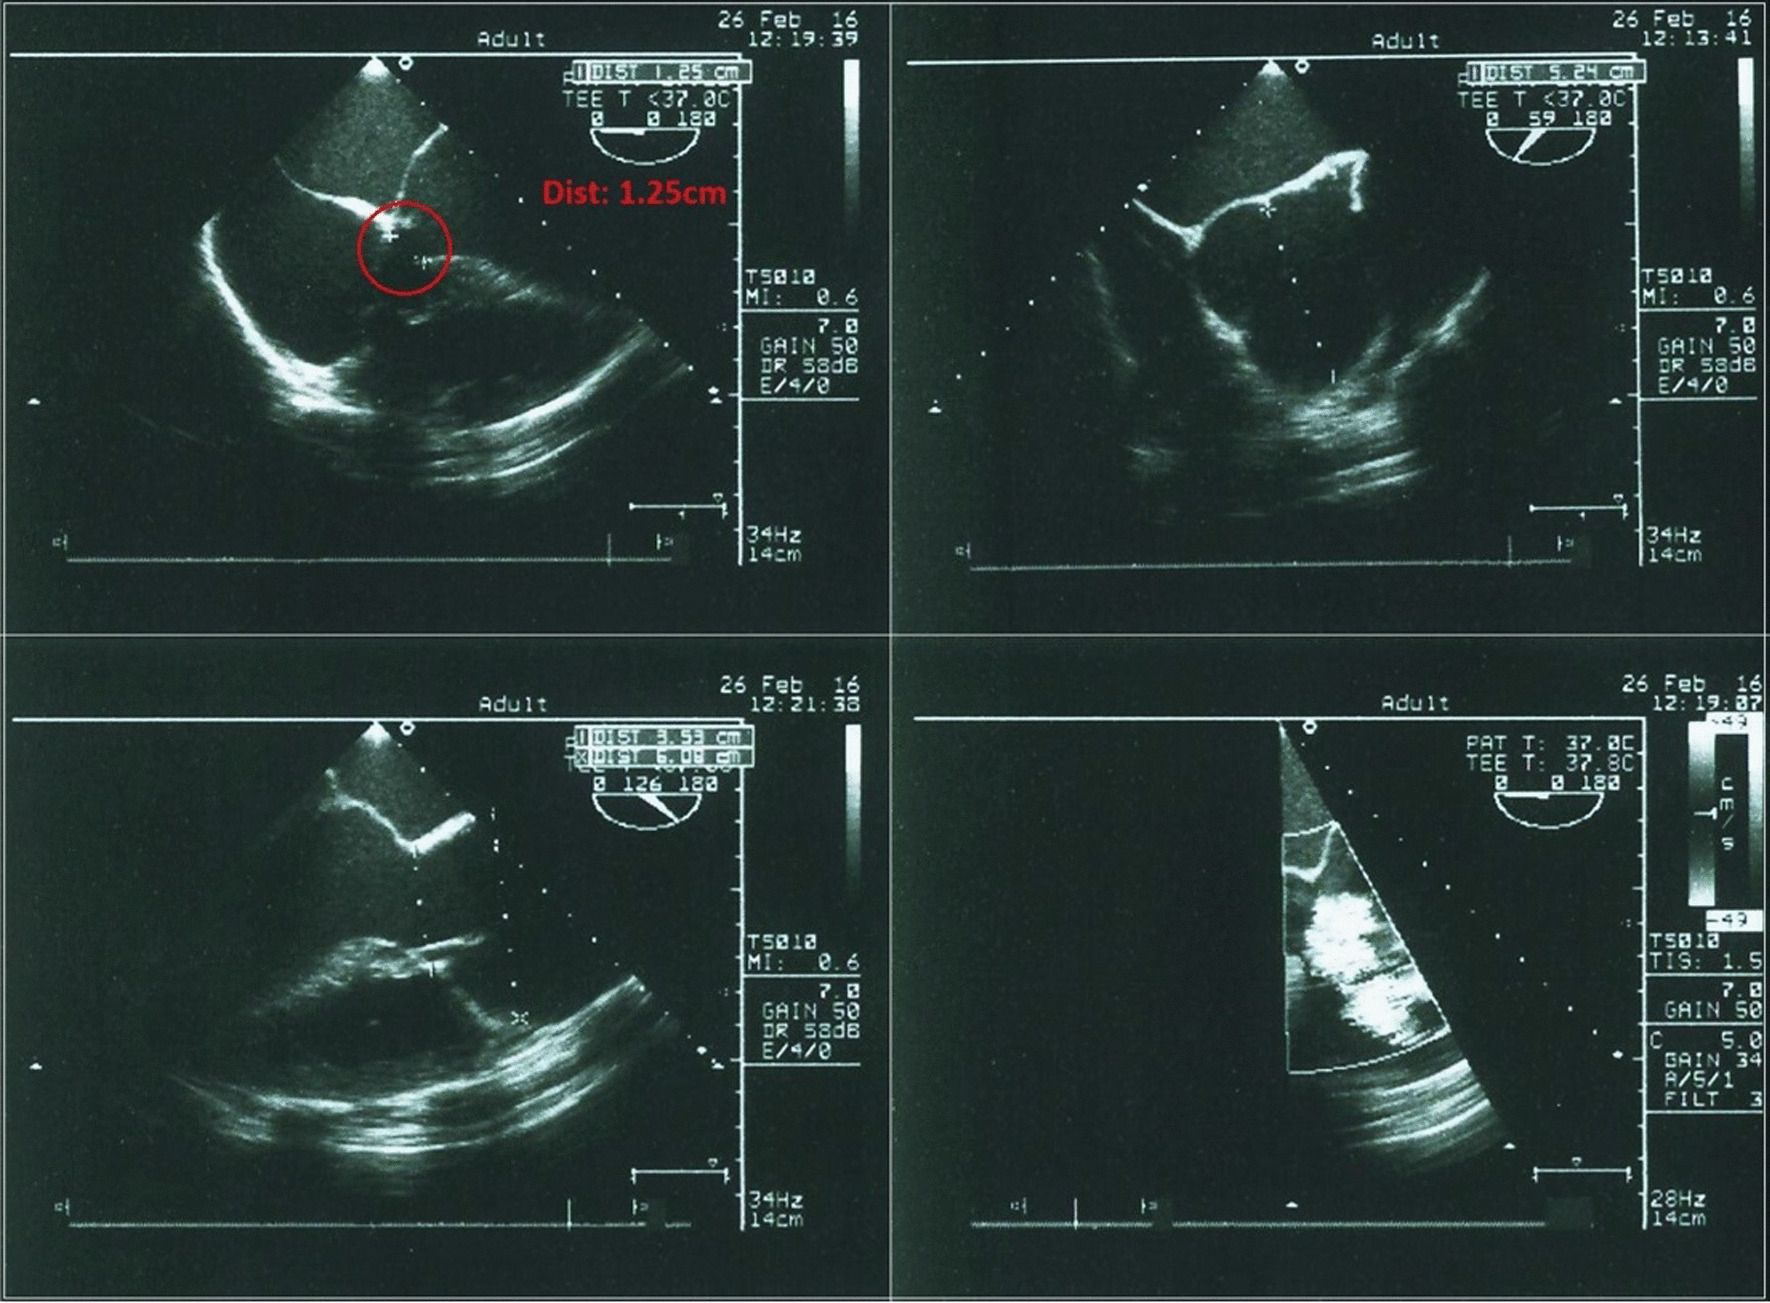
Transesophageal echocardiography views
